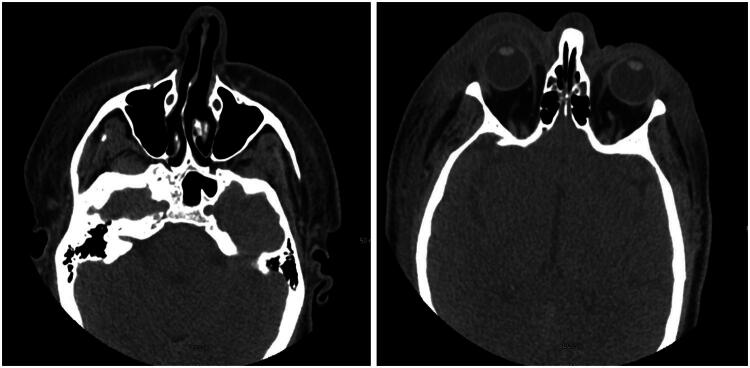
CT maxillofacial scan with findings concerning for a subcutaneous soft tissue infection.
